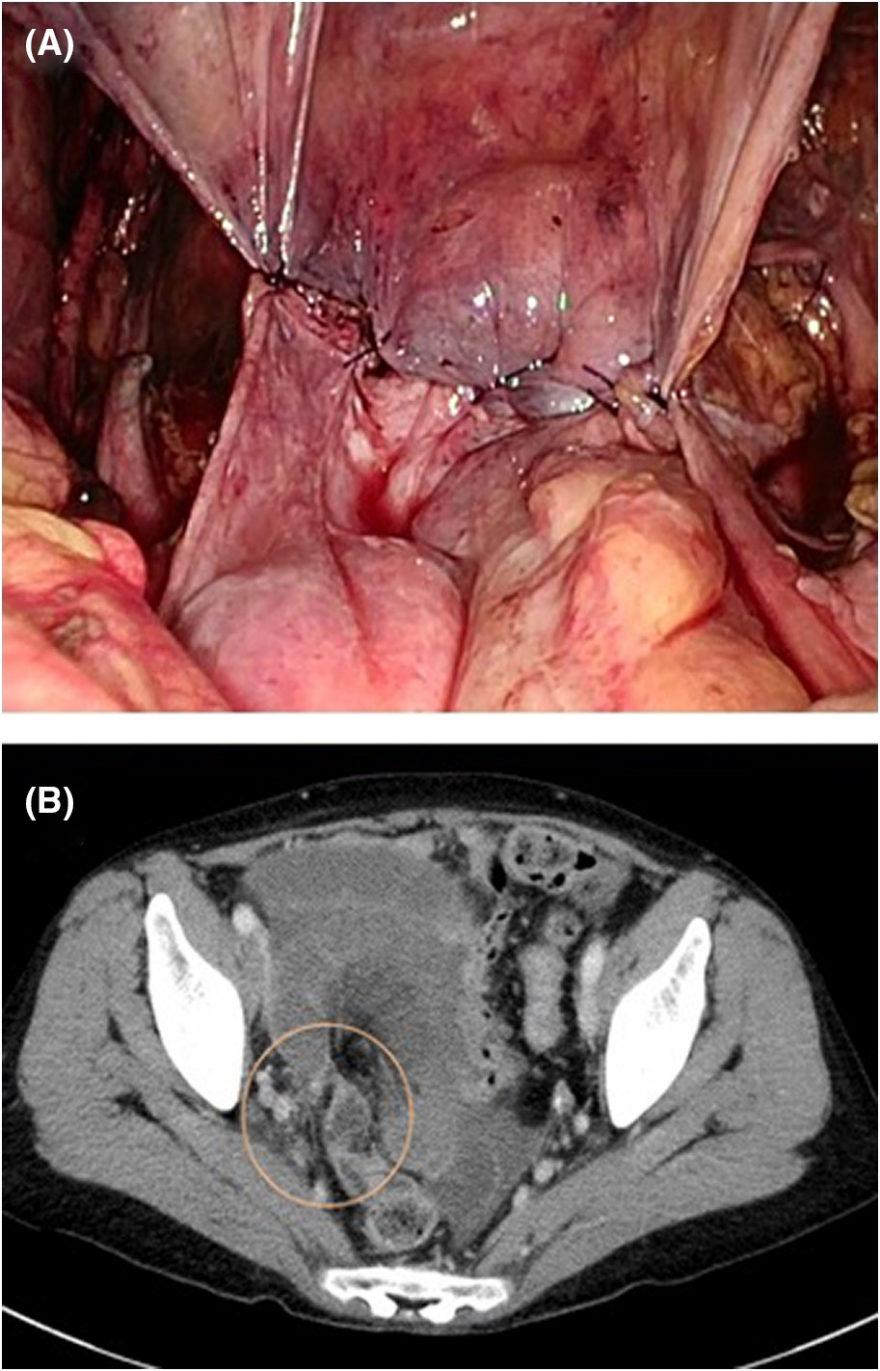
(A) The status of the pelvic peritoneum after the first surgery. (B) Contrast‐enhanced computed tomography scan revealed a closed ileal loop in the right pelvic cavity. The ileum wall showed ischemic change and the mesentery was edematous.
